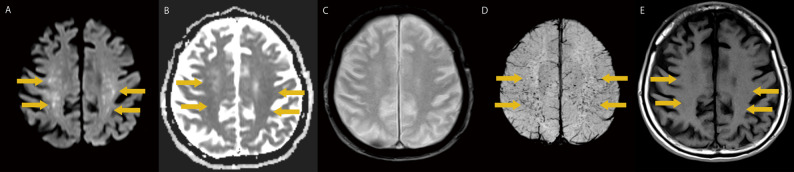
Brain MRI obtained 7 days after symptom onset demonstrating characteristic findings of cerebral fat embolism and superior sensitivity of SWI. A DWI shows multiple small hyperintense lesions (arrows) distributed bilaterally in the cerebral white matter representing cytotoxic edema caused by fat emboli. The lesions demonstrate the characteristic "starfield pattern" with multifocal distribution crossing multiple arterial territories. B ADC map at a representative level shows restricted diffusion (hypointense signal, arrows) in the affected areas, confirming acute cytotoxic edema. C Conventional T2*-weighted imaging at a representative level shows no detectable microhemorrhages, appearing essentially normal. D SWI at a representative level reveals numerous hypointense lesions (arrows) representing microhemorrhages, with the characteristic distribution pattern involving the cerebral white matter. Note the marked discrepancy between T2* (panel C, negative) and SWI (panel D, extensive abnormalities), demonstrating the superior sensitivity of SWI for detecting cerebral microhemorrhages in fat embolism. E Pre-contrast T1-weighted imaging at a representative level shows subtle hypointensity (arrows) in regions corresponding to the DWI hyperintensities (panel A). No T1 hyperintensity is observed, indicating that individual fat globules are too small to be directly visualized despite their presence as evidenced by microhemorrhages on SWI (panel D)
